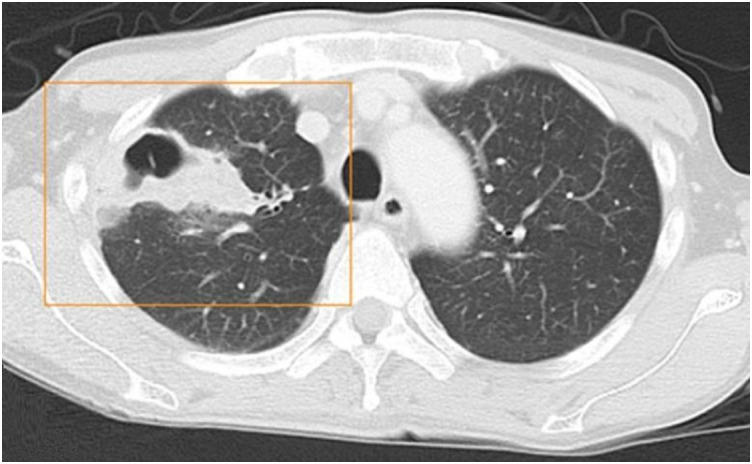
Chest computed tomography showed a 2.6-cm cavitary lesion in the right upper lobe with bilateral pulmonary infiltrates.
